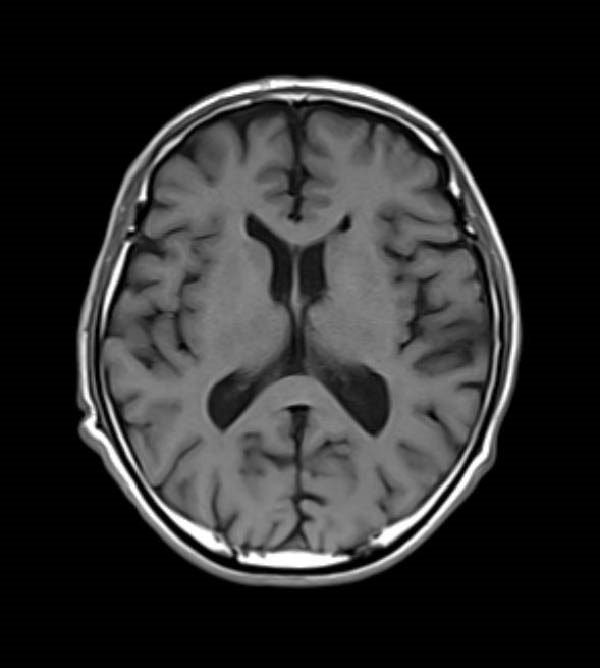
Magnetic resonance imaging (MRI) revealed no significant brain atrophy. MRI was performed with a 1.5‐T scanner (Siemens Healthineers) using a T1‐weighted sequence with the following parameters: repetition time = 450 ms; echo time, 12 ms; field of view, 207 × 229 mm²; matrix size, 319 × 288; slice thickness = 5.0 mm.
